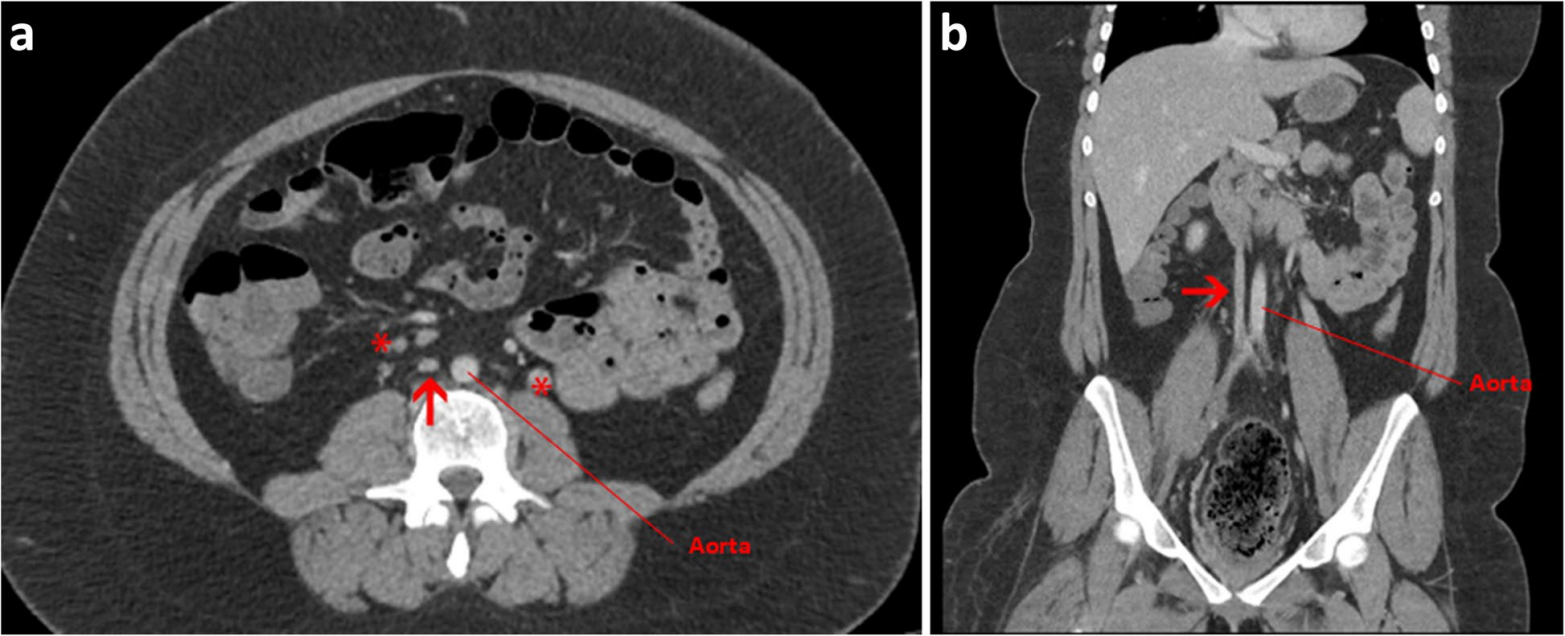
a Axial CT venogram shows small calibre IVC over a long distance (arrow) and established collateral veins (asterisks) compatible with hypoplastic IVC. b Coronal CT venogram shows small calibre IVC over a long distance (arrow) compatible with hypoplastic IVC
